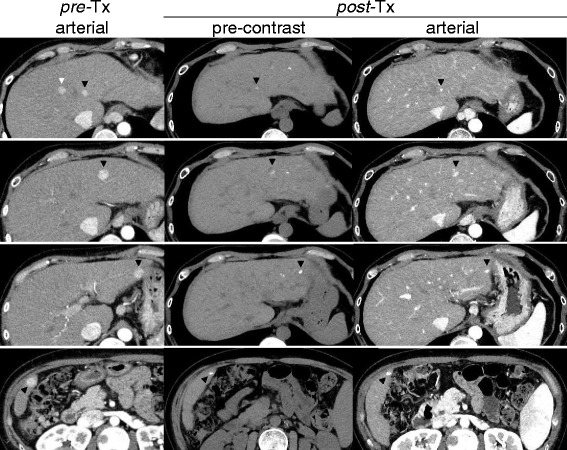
Representative computed tomography images from the study arm (case 4 in Table 4) treated with the miriplatin-TOCE/DDP-H-HAIC combination therapy. *Pre-*TX arterial: Multiple nodules were spread in both lobes and enhanced in the late arterial phase of a dynamic computed tomography study performed prior to the treatment (*black* and *white arrowheads*). *post*-Tx pre-contrast: These nodules were detected as high density spots of lipiodol without an injection of contrast medium three months after the eighth session of combination therapy (*black arrowheads*). *post*-Tx arterial: The high density spots were scarcely enhanced and markedly reduced in size (*black arrowheads*). One nodule, which is indicated by the *white arrowhead* in the *pre-*Tx arterial column, could not be detected at post-Tx either in the pre-contrast or in the arterial phase
